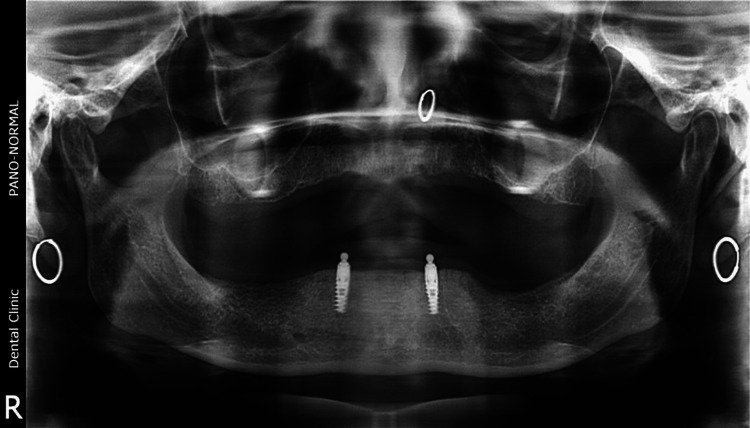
Orthopantomogram after implant placement
